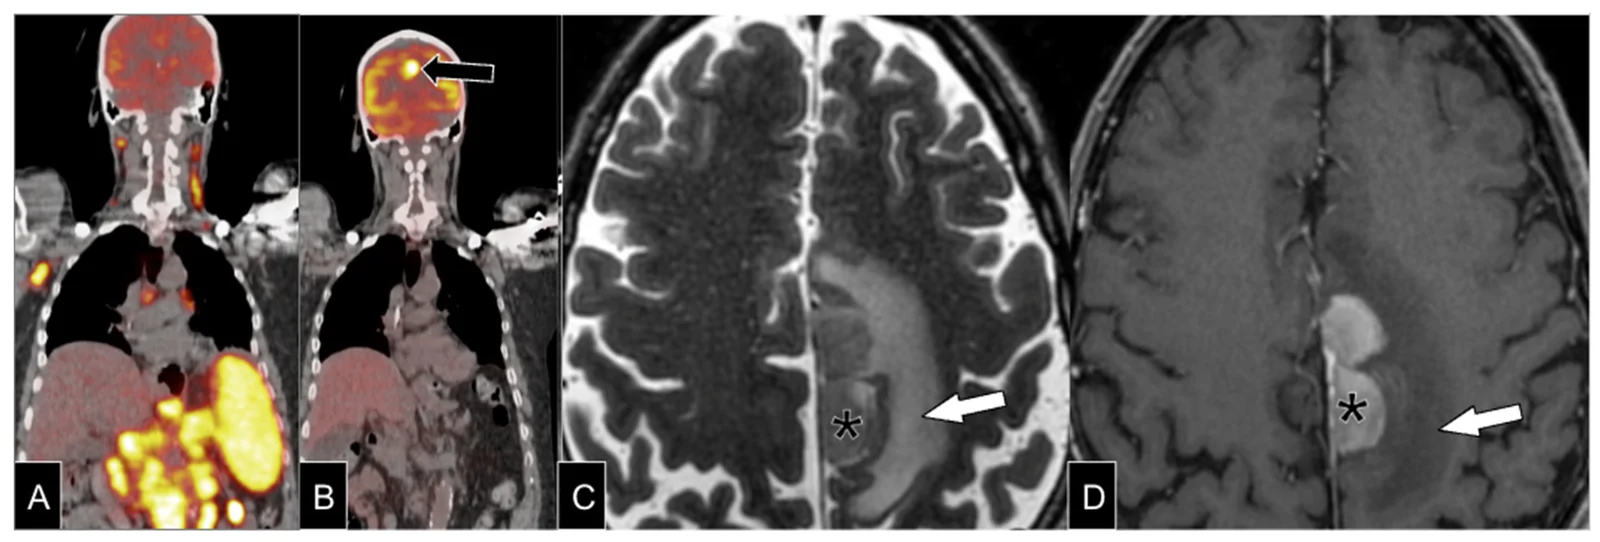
Systemic lymphoma and CNS relapse. Baseline FDG-PET/CT (A) shows increased uptake in multiple lymph node chains and spleen, without CNS involvement. Six-month follow-up post-treatment FDG-PET/CT (B) shows remission of abdominal disease, with a new brain lesion (black arrow). Axial T2-weighted (C) and contrast enhanced T1-weighted (D) MR images show a parenchymatous expansive lesion (asterisks) with low T2 signal intensity and homogeneous contrast enhancement. Also note prominent perilesional edema (white arrow).
